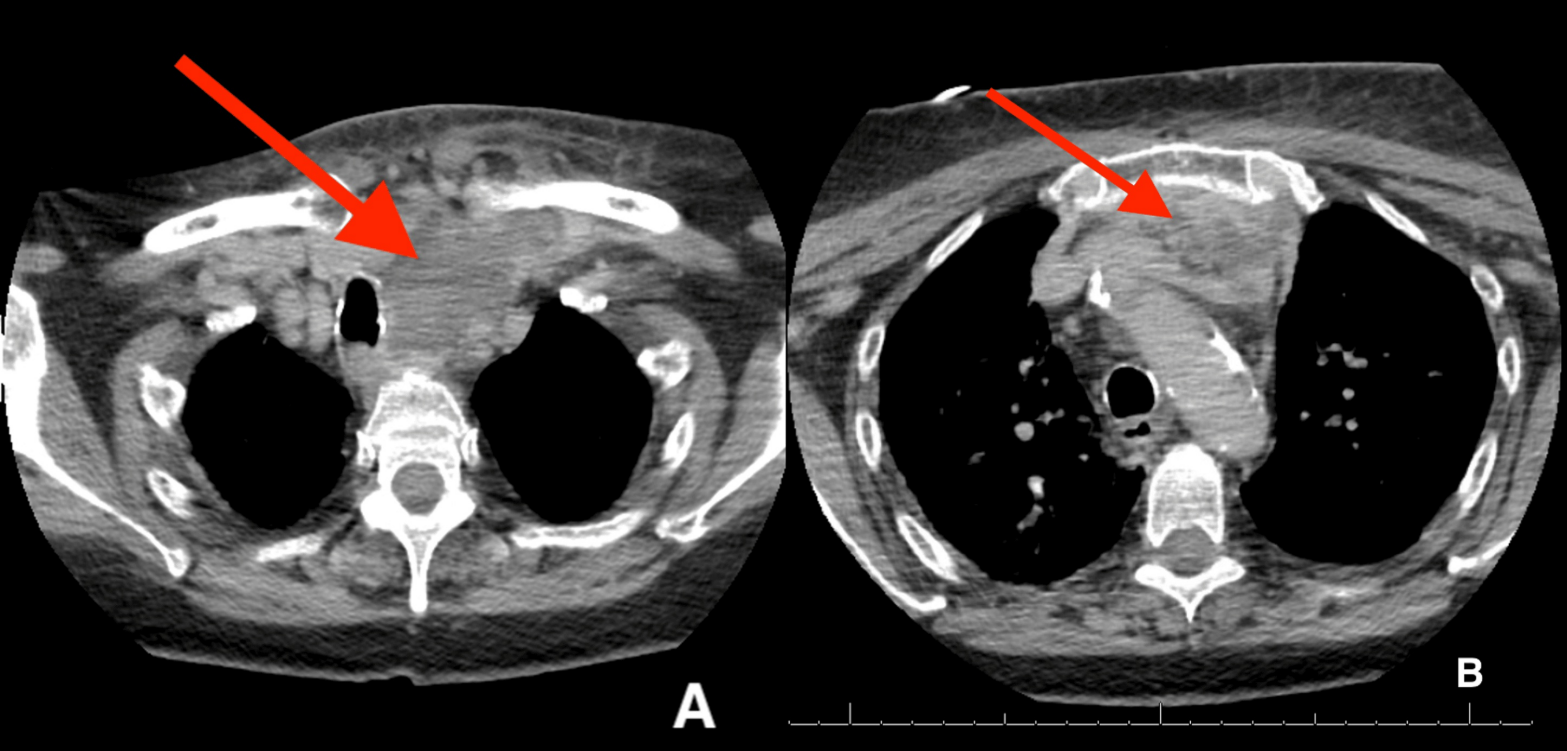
Neck CT with contrast CT: Computed tomography A and B showing two axial planes of the patient's neck CT with contrast. Arrows indicate a multiseptated collection in the left base of the neck, consistent with an abscess measuring approximately 7.0 x 4.5 x 5.4 cm. A shows the superior axial view of the abscess causing mass effect on the trachea 1.5 cm to the right of midline. B shows an inferior axial view of the abscess as it extends to the level of the aortic arch
